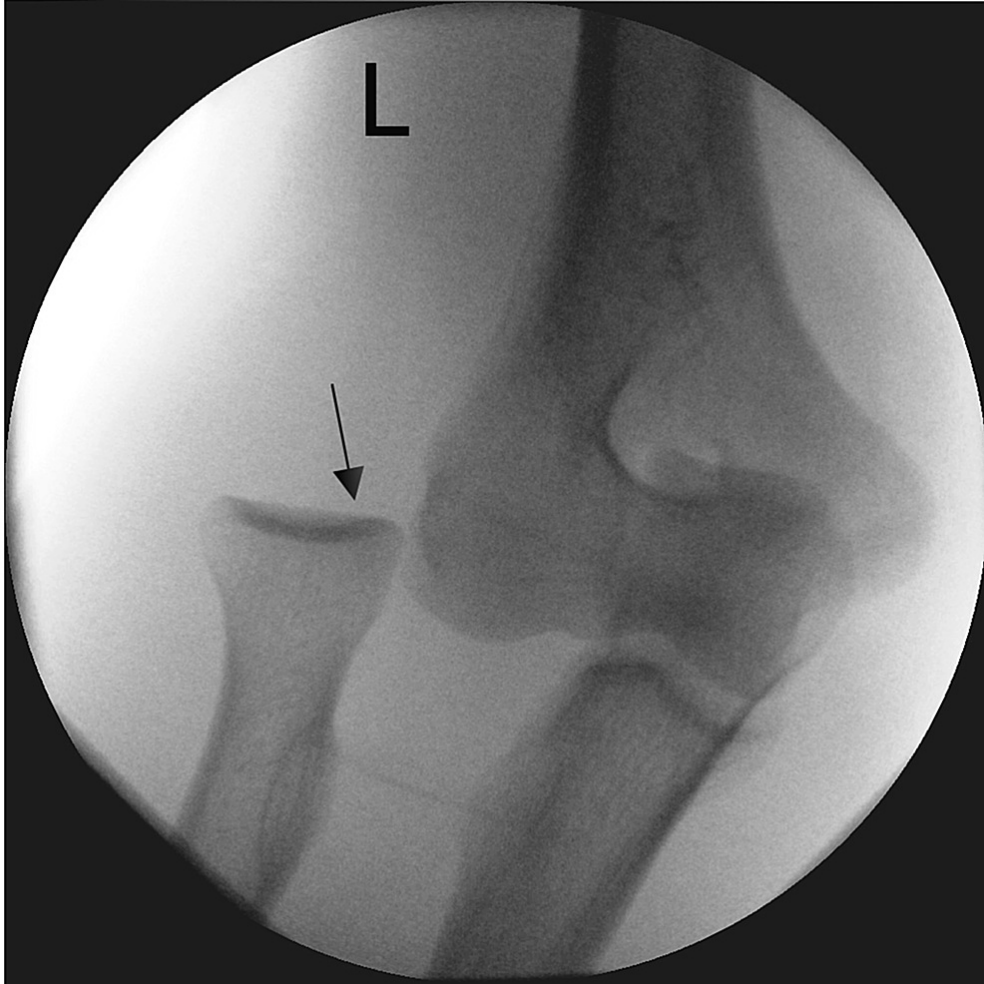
Perioperative X-rays: lateral dislocation of the radial head Lateral dislocation of the radial head combined with a concomitant injury is rarely seen.
